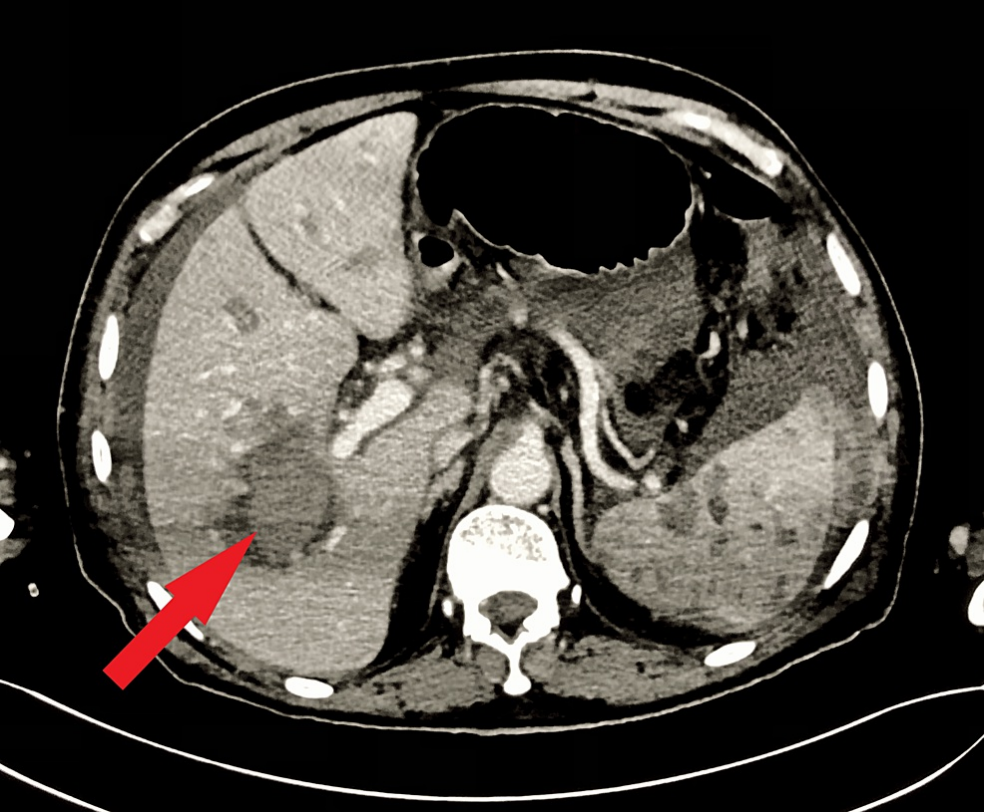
Axial computed tomography (CT) scan of the abdomen revealing peliosis hepatis (arrow)
